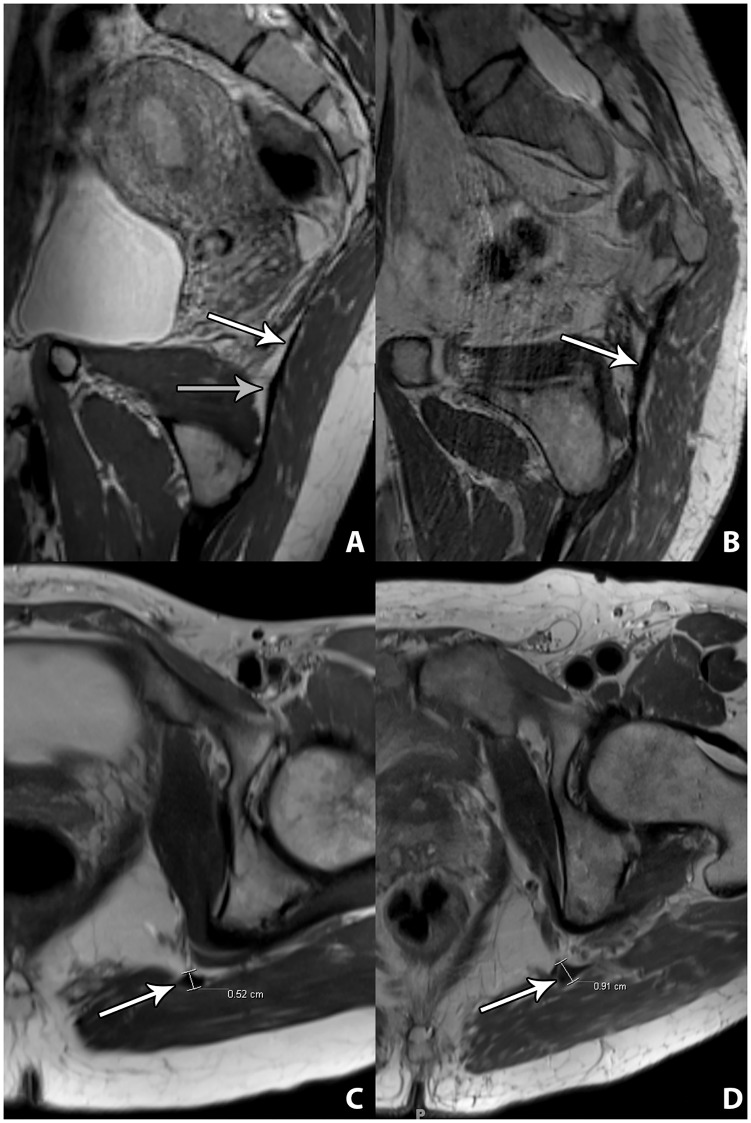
Three-dimensional 3-Tesla MR Neurography of the healed sacrotuberous ligament following complete division and grafting. A: Sagittal oblique multiplanar intermediate-weighted MR image demonstrates continuity of the healed sacrotuberous ligament (STL) (white arrow) after previous complete division, indicative of spontaneous healing. The gray arrow indicates the healed division site. B: Sagittal oblique multiplanar intermediate-weighted MR images demonstrate continuity of a STL allograft (white arrow). C: Two-dimensional 3-Tesla MR Neurography of the healed STL following complete division and grafting, at level of Alcock’s canal. Axial intermediate-weighted MR image demonstrates the anteroposterior dimensions of the healed STL (white arrow) after previous complete division. D: Axial intermediate-weighted MR image the anteroposterior dimensions of the STL allograft (white arrow).
